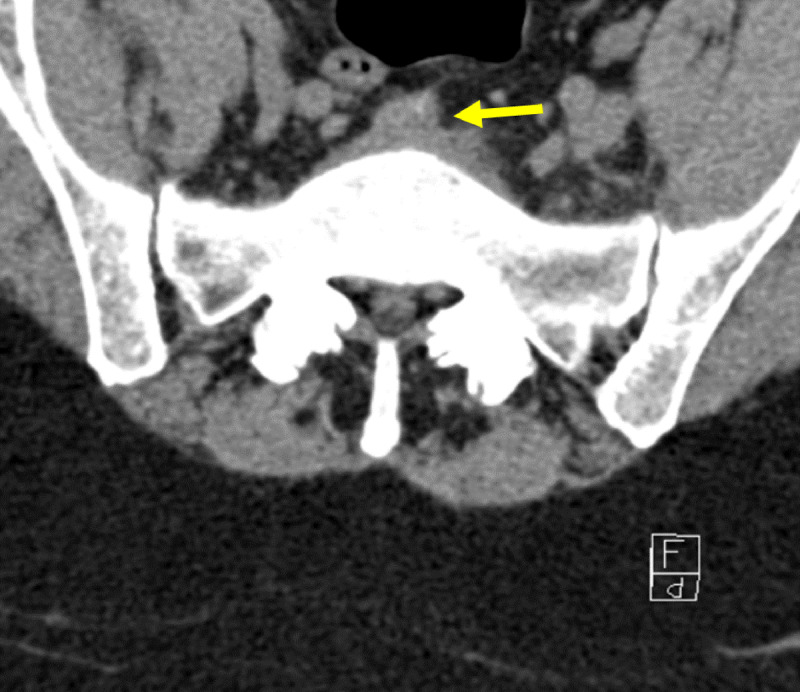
Transverse non-contrast CT of L5-S1 disk: Initial CT-scan showing collection in front of disk.
